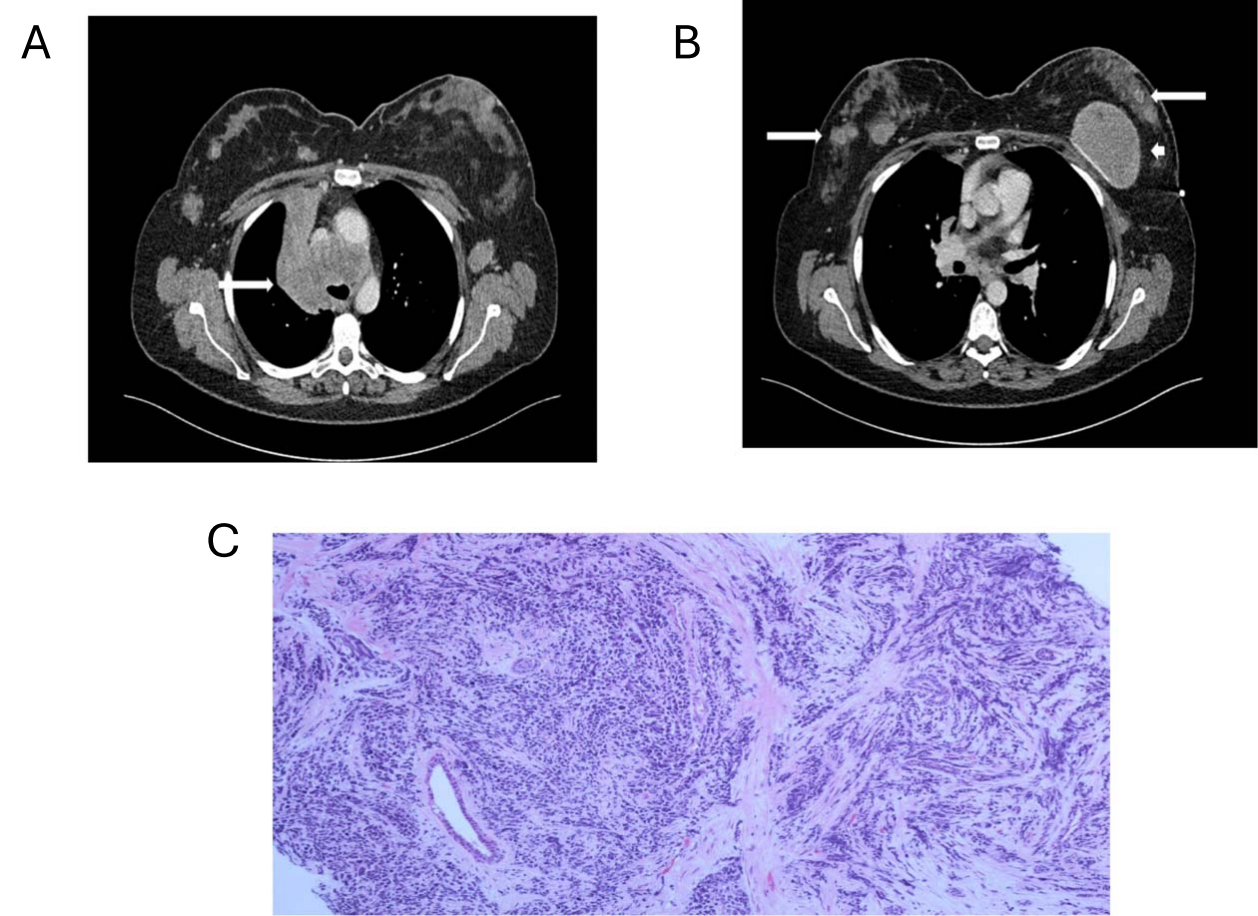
(A) Axial contrast enhanced CT shows right hilar mass. (B) CT scan showing bilateral breast metastasis (arrow) and left breast implant (arrowhead). (C) Cores of breast tissue infiltrated by malignant cells with high nuclear: cytoplasmic ratio, inconspicuous nucleoli and nuclear molding. The appearances are of small cell carcinoma.
